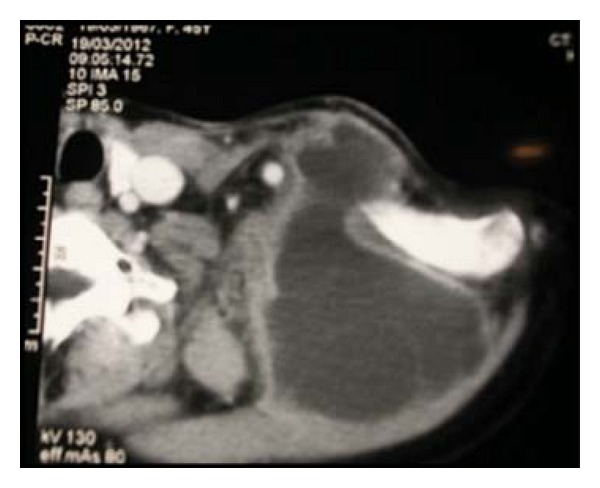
CT scan demonstrated cystic structure in the left supraclavicular region with multivesicular lesions.
